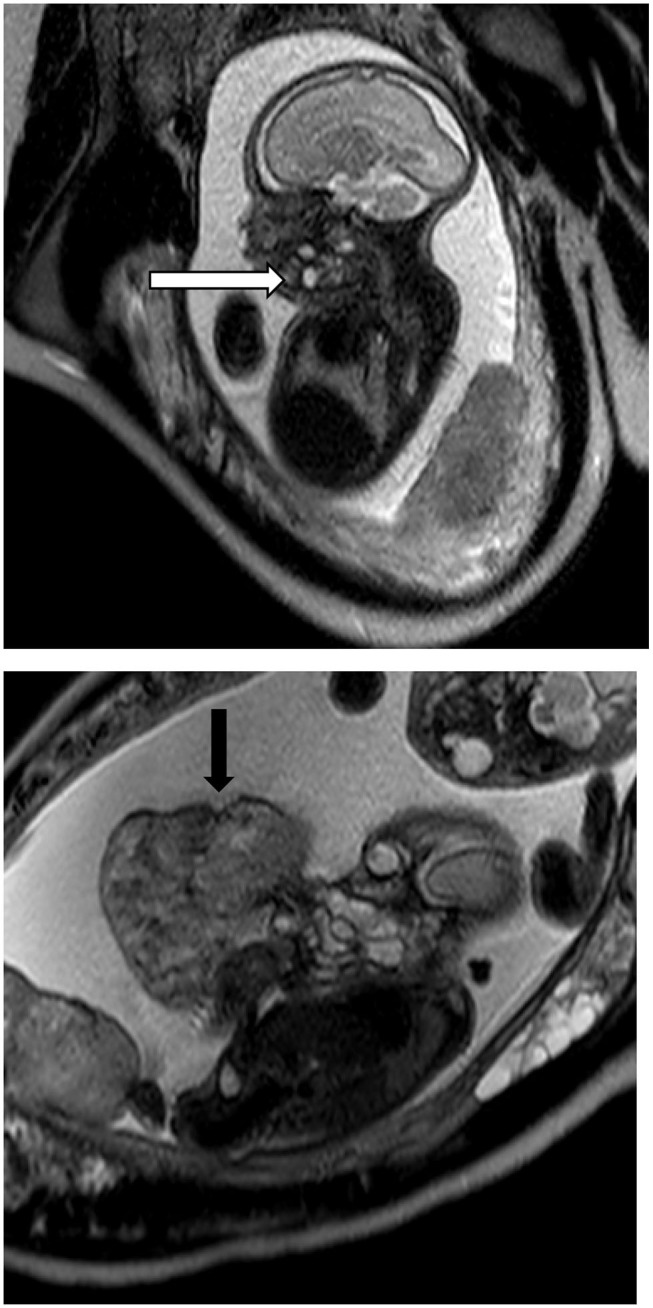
Fetal MRI of Twin A and Twin B. Twin A demonstrates a solid and cystic lesion at the floor of the mouth with obliteration of the oropharyngeal cavity (white arrow) while Twin B has the large epignathic teratoma arising from the oral cavity (black arrow).
